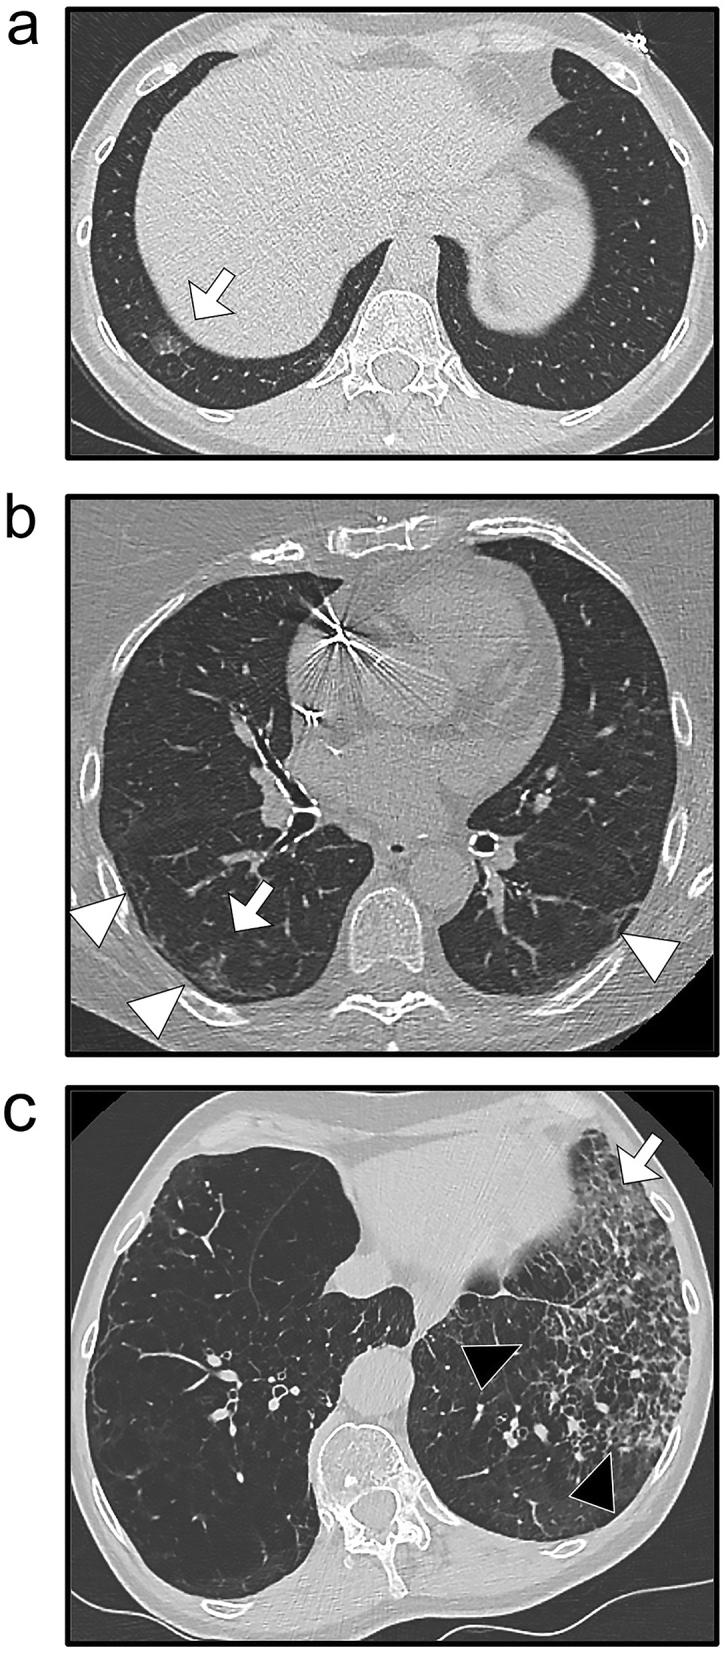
Added value of the final clinical-radiological model for patients with indeterminate/compatible radiological conclusions. (a) A 50-year-old woman presented at the emergency with chest pain, fever, and cough for less than one week. Chest CT showed a basal-predominant peripheral ground-glass opacity (GGO—white arrow) in the lower right lobe. The probability for RT-PCR + was 68.9%. (b) A 71-year-old woman presented at the emergency with cough, dyspnoea, fever and asthenia for 1–2 weeks. Chest CT showed bilateral peripheral fibrotic bands (white arrowheads) with a peripheral right GGO (white arrow). The probability for RT-PCR + was 65%. (c) A 61-year-old woman with a medical history of active smoking, emphysema and chronic obstructive pulmonary disease presented at the emergency with a cough, dyspnoea, fever and asthenia. Chest CT showed peripheral predominant intralobular reticulations in the lower left lobe (black arrowheads) with a single area of non-rounded GGO (white arrow). The probability for RT-PCR + was 57.9%. In the three cases, the RT-PCR was indeed positive.
